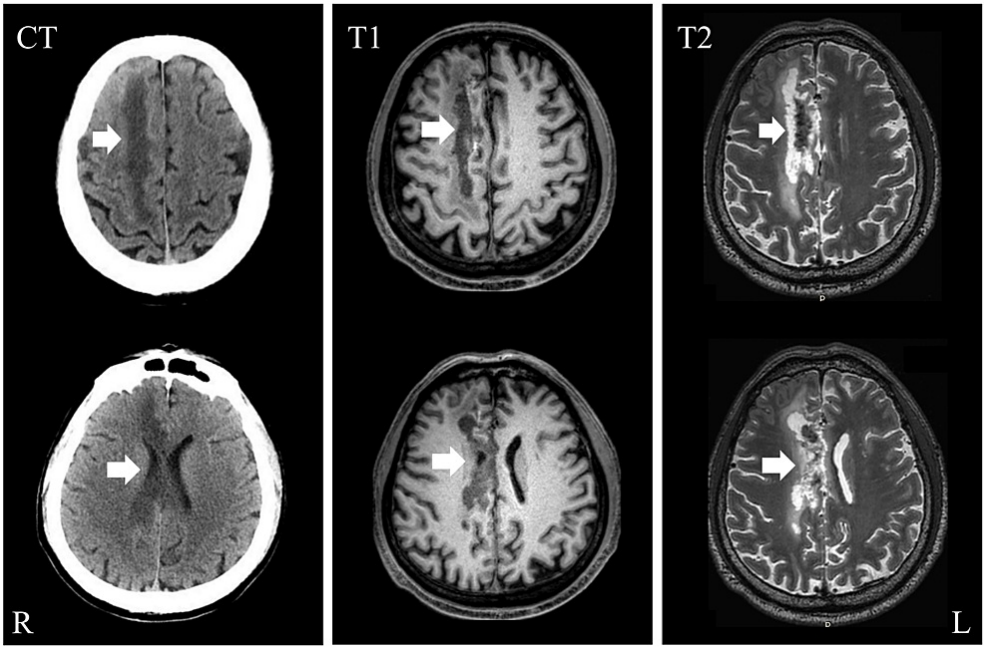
CT and MRI (T1&T2) imaging. The computed tomography scan revealed the presence of infarctions in the right parietal lobe and corpus callosum, accompanied by a small local hemorrhagic lesion. The MRI findings showed infarctions in the right centrum semiovale and genu of corpus callosum, as well as bilateral subcortical lacunar infarctions.
